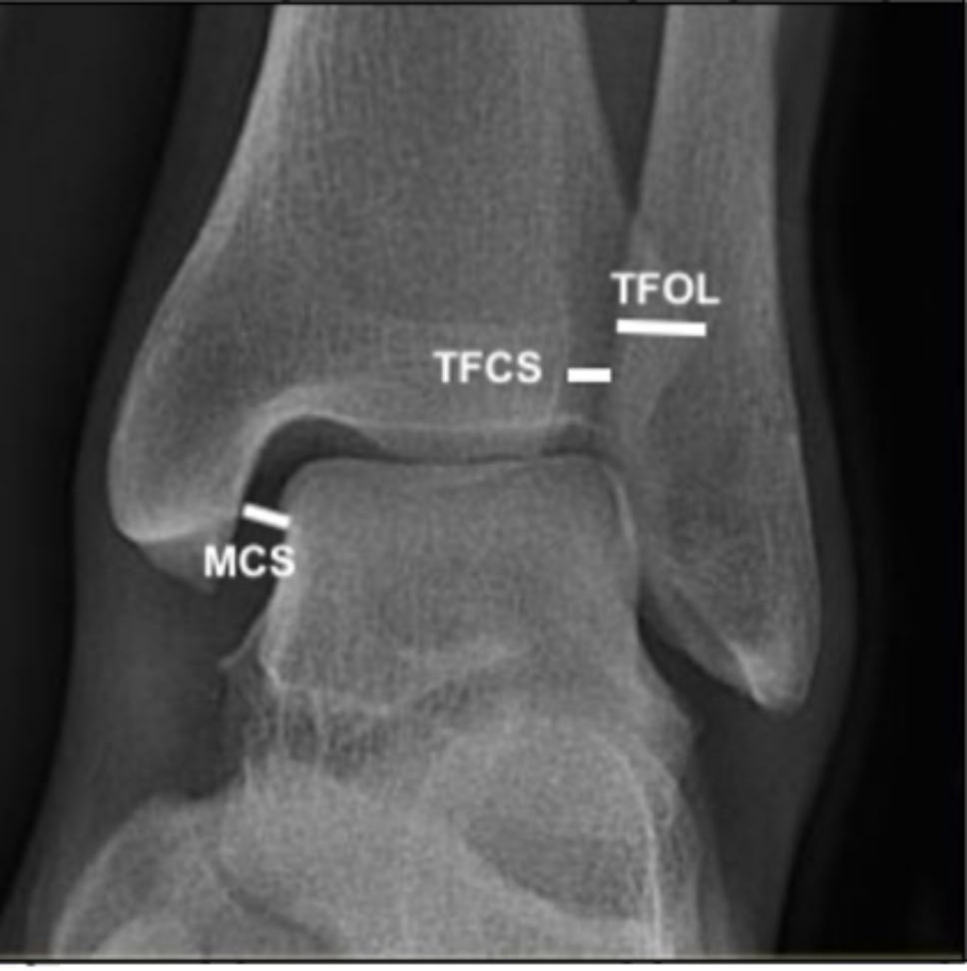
Radiographic AP view of the ankle joint showing the tibiofibular clear space (TFCS), the medial clear space (MCS), and the tibiofibular overlap (TFOL)
